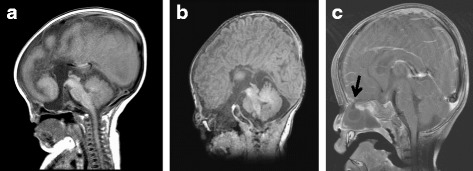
Brain magnetic resonance imaging scans of the patients with congenital basal meningocele. Sagittal T1-weighted images indicated transethmoidal meningocele and agenesis of the corpus callosum in patient 1 (a), sphenoethmoidal meningocele and agenesis of the corpus callosum in patient 2 (b), and transethmoidal meningocele (arrow) in patient 3 (c)
